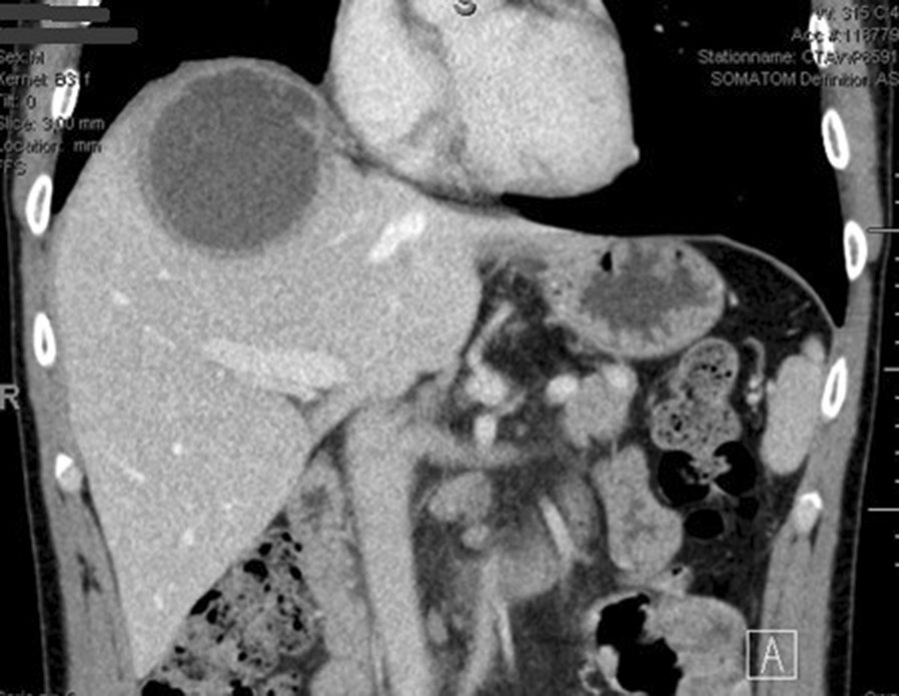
Upper abdominal computed tomography scan revealing an abscess of 7 cm in the right liver lobe with a small margin of liver tissue to the right hemidiaphragm and subcapsular extension
